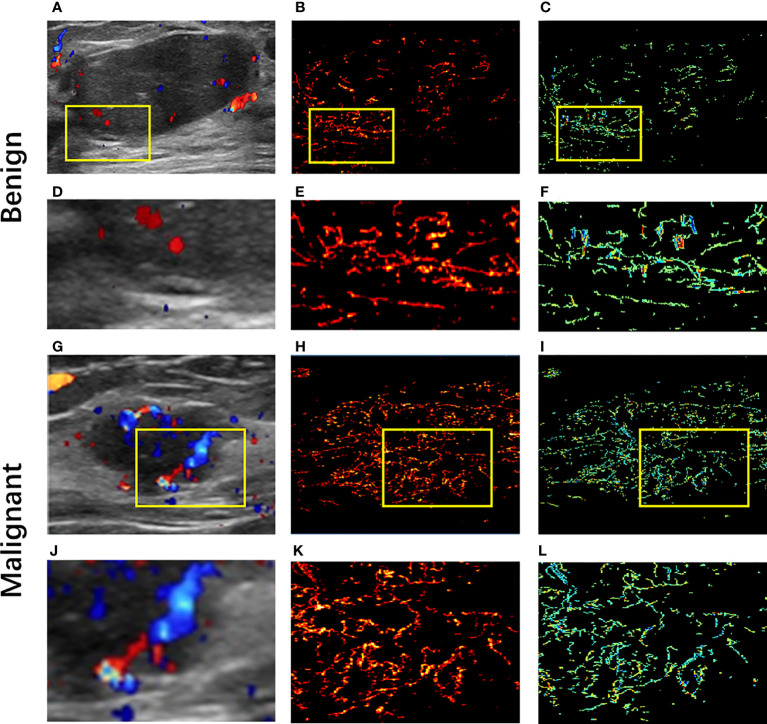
Ultrasound images of benign and malignant breast masses respectively. (A, G) Color-Doppler flow images of benign and malignant breast masses respectively; (B, K) super-resolution images of benign and malignant breast masses respectively; (C, I) super-resolved velocity images of benign and malignant breast masses respectively. (D–F) Zoomed-in sections as the yellow box indicated in (A–C); (J–L) Zoomed-in sections as the yellow box indicated in (G–I).
